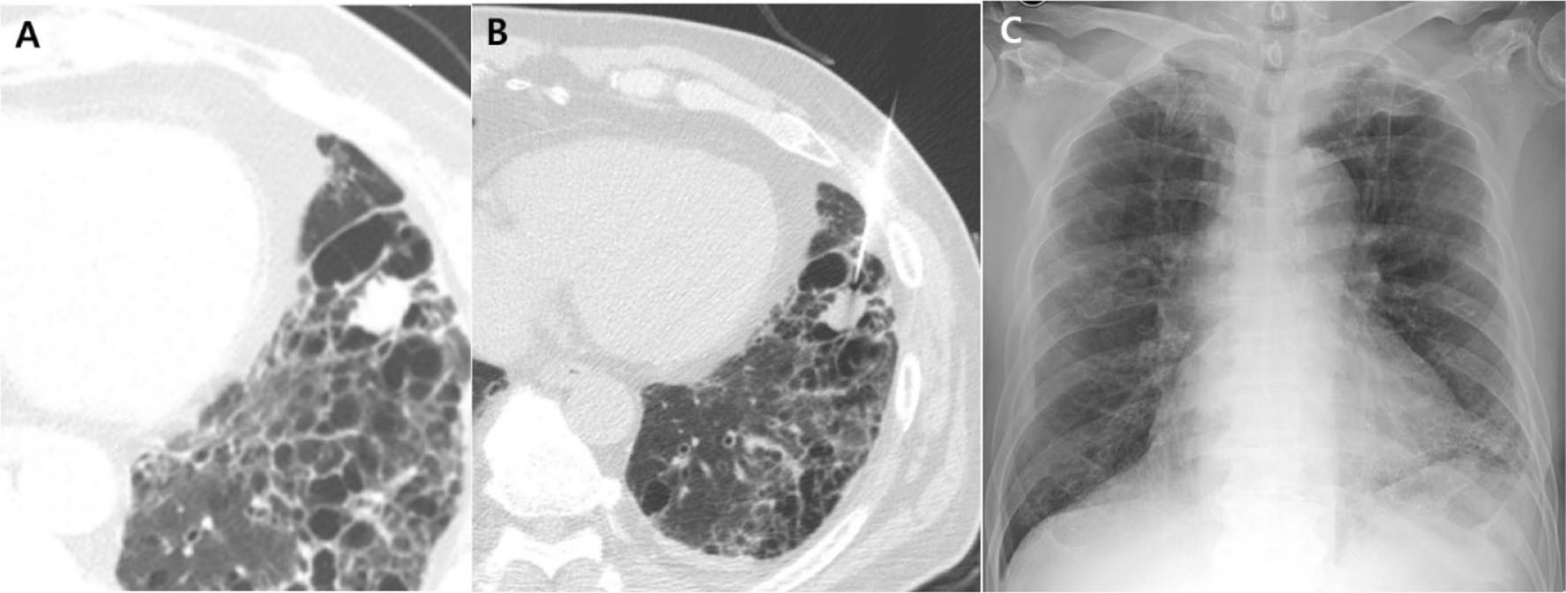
A 67-year-old male patient with underlying idiopathic pulmonary fibrosis. The patient showed a 1.6-cm irregular nodule in his left lower lobe (A). CT-guided percutaneous core needle biopsy (PCNB) was performed with the patient in the supine position. The needle traversed the honeycomb cysts (B). There was no complication after PCNB (C). Squamous cell carcinoma was confirmed through PCNB.
